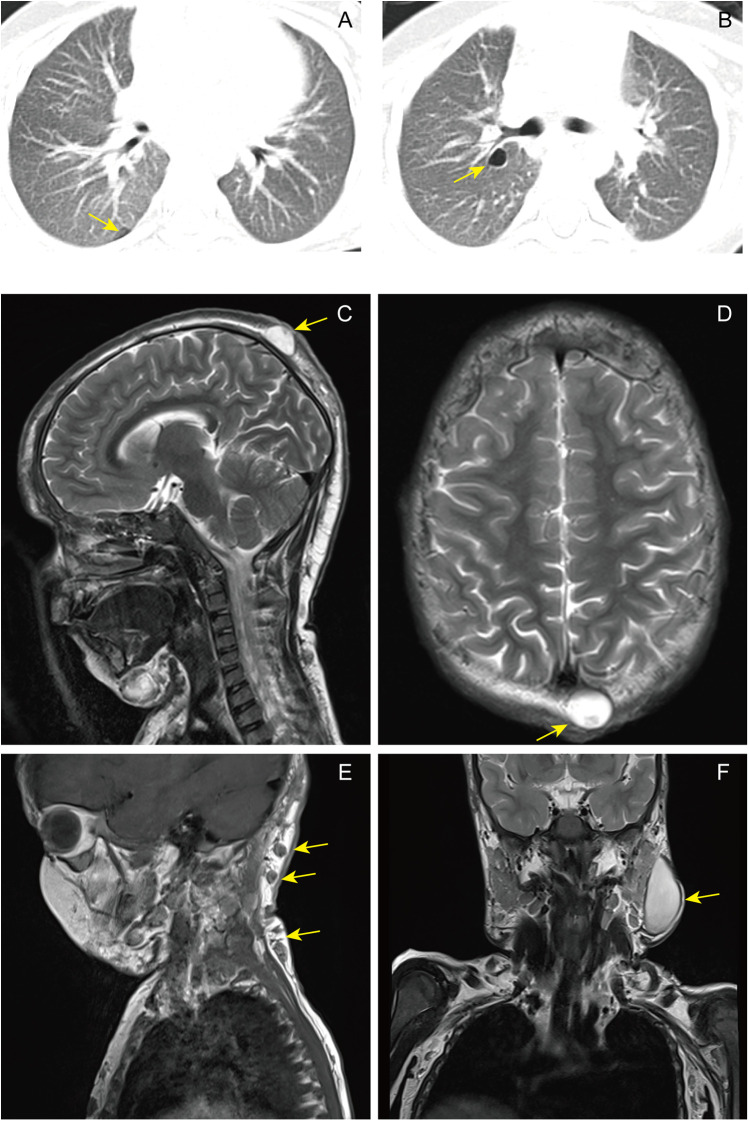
Radiological findings of pulmonary and subcutaneous manifestations in this case. (A,B) Axial chest CT images (lung window) demonstrate characteristic pulmonary pneumatoceles. (A) A localized hyperlucent area is visible in the right lower lobe posterior basal segment. (B) A round, thin-walled, well-defined air cyst with an absence of lung markings is present in the right lower lobe dorsal segment. (C–F) Magnetic resonance imaging (MRI) reveals subcutaneous “cold” abscesses in the head and neck region. (C) Sagittal T2-weighted image shows a well-circumscribed, round hyperintense lesion in the left parietal scalp. (D) Axial T2-weighted image confirms the round, hyperintense lesion in the left parietal subcutaneous tissue. (E) Sagittal T1-weighted image demonstrates multiple round, isointense lesions in the occipitocervical subcutaneous tissue. (F) Coronal T2-weighted image shows an oval, hyperintense (long T2) lesion in the left lower jaw area subcutaneous tissue.
